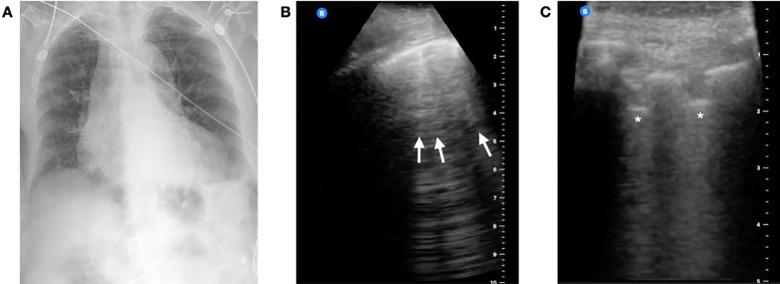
Case 1 imaging findings: (A) Anterior-posterior chest radiograph was read as negative, (B) Lung point-of-care ultrasound revealed B-lines anteriorly (arrows), (C) and an irregular pleural line with subpleural consolidations posteriorly (asterisks).
